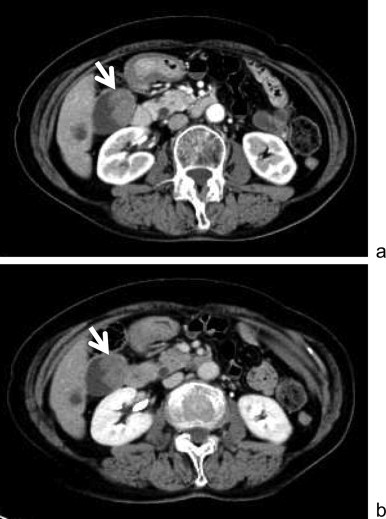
Contrast abdominal CT showed an early enhanced mass in the gallbladder (arrow) and the enhancement persisted on the equilibrium phase, suggesting gallbladder cancer. (a) Early phase. (b) Equilibrium phase.
